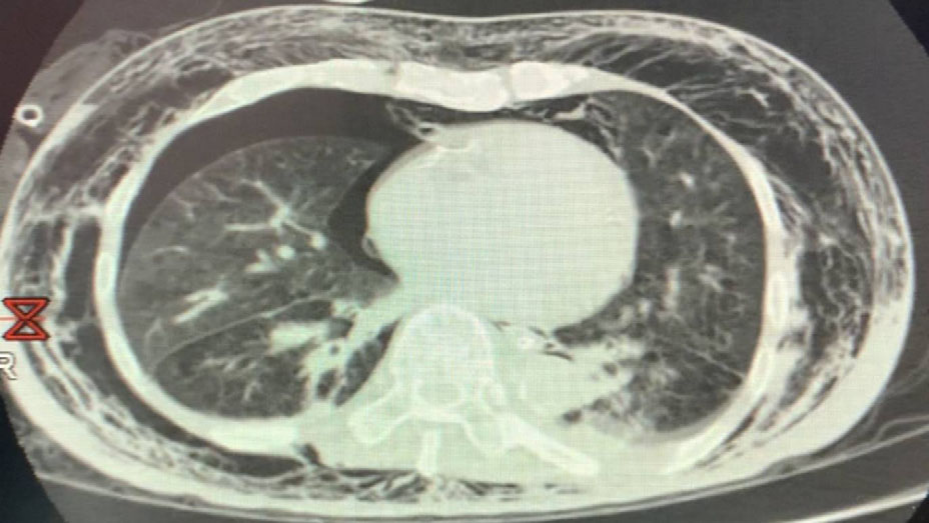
Computed tomography (CT) of the thorax demonstrating extensive subcutaneous emphysema together with right pneumothorax and pneumomediastinum.
